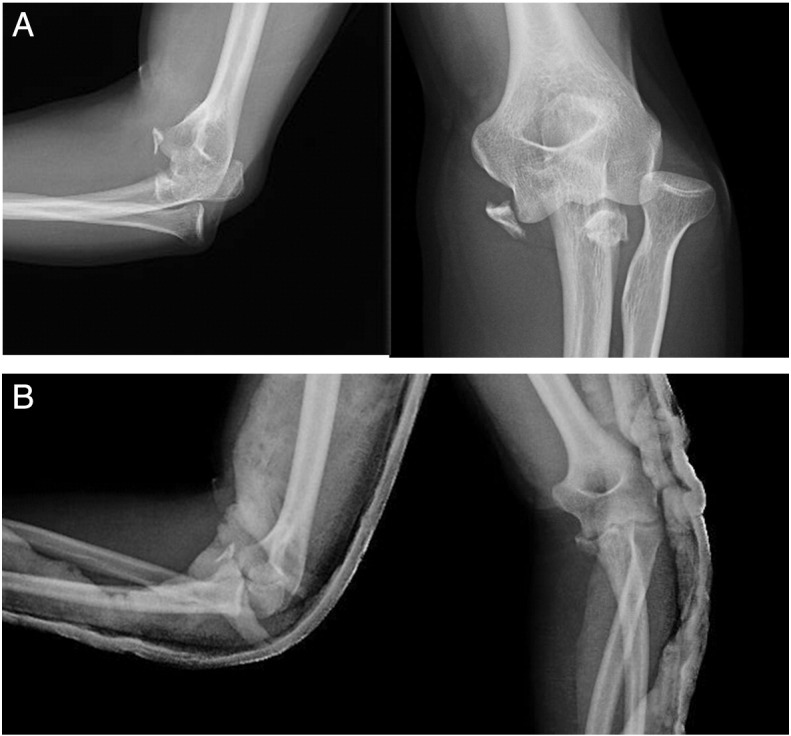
(A–B) Initial radiographic examination shows a posterolateral elbow dislocation and a fracture of the anteromedial coronoid (A). After reduction, a CT scan with 3D reconstruction was performed which better delineates the anteromedial fracture fragment and an associated anterolateral fracture fragment (B).
